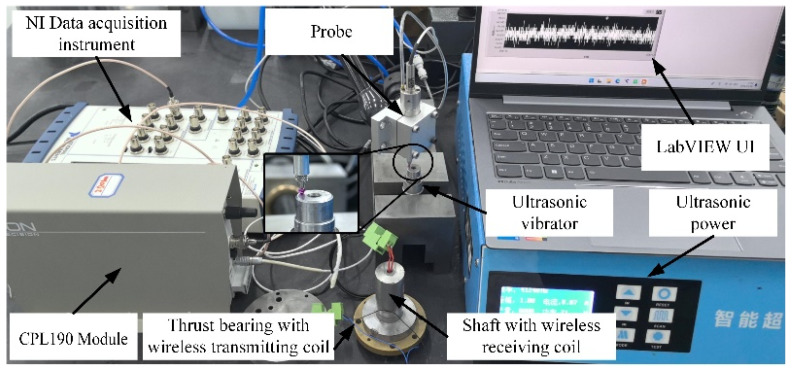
Ultrasonic transducer testing setup.
